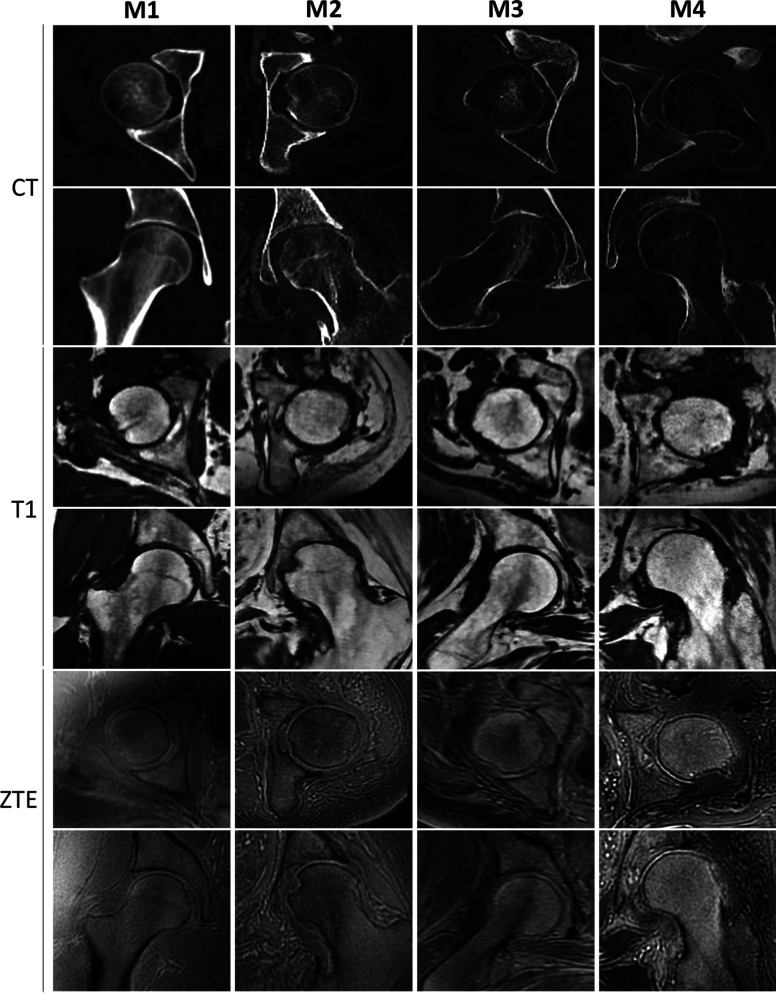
Osseous contour resolution as seen on CT scans, T1-weighted MRI scans, and ZTE MRI scans. (Reproduced from: Amar R, Thiry T, Salga M, Safa D, Chetrit A, Gatin L, Carlier RY. Comparison of magnetic resonance imaging and computed tomography for bone assessment of neurogenic heterotopic ossification of the hip: a preliminary study. J Orthop Surg Res. 2021 Dec 20;16[1]:725^27^, under a Creative Commons Attribution 4.0 International License.)
